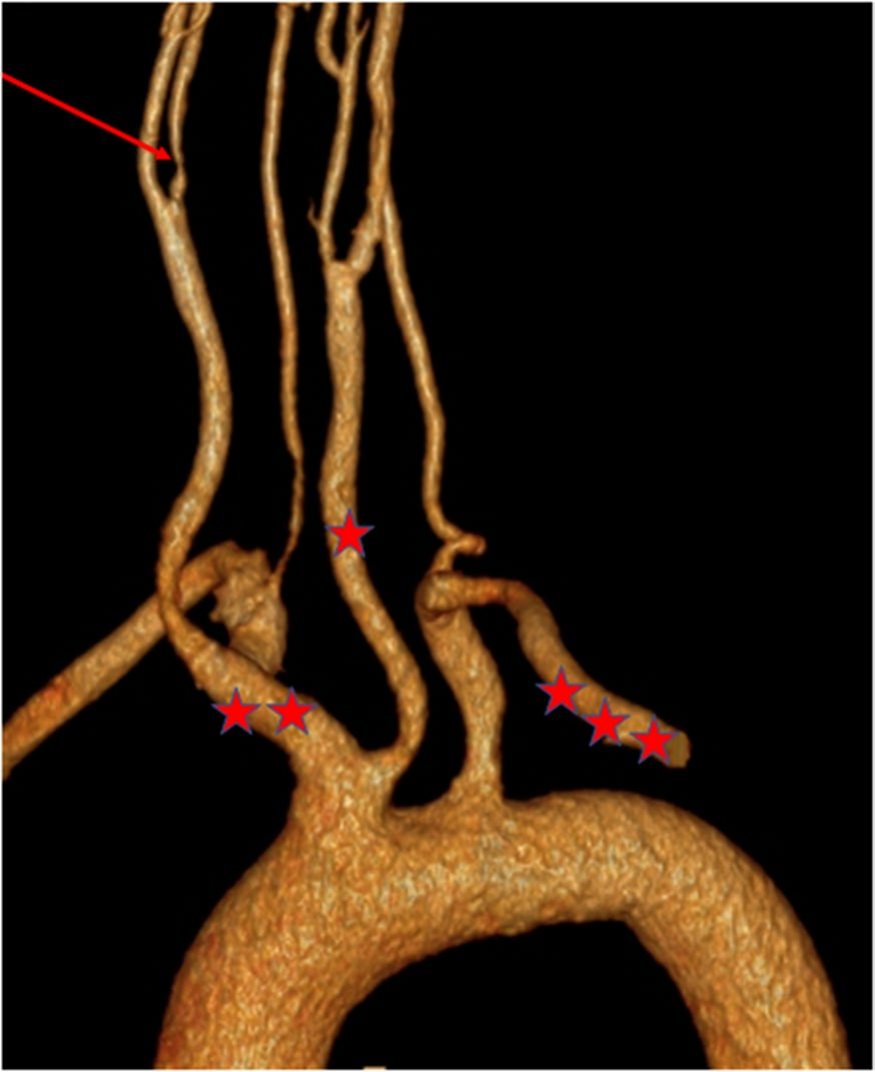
The supra aortic branches of the aorta are shown in the 3D reconstruction of CT angiography. A red asterisk signs the brachiocephalic trunk; it branches to the right common carotid artery and the subclavian artery. The red arrow shows the significant stenosis of the right internal carotid artery distal from the carotid bifurcation. Two red asterisks sign the left common carotid artery. Three red asterisks sign the left subclavian artery second part distal form the origin of the left vertebral artery
